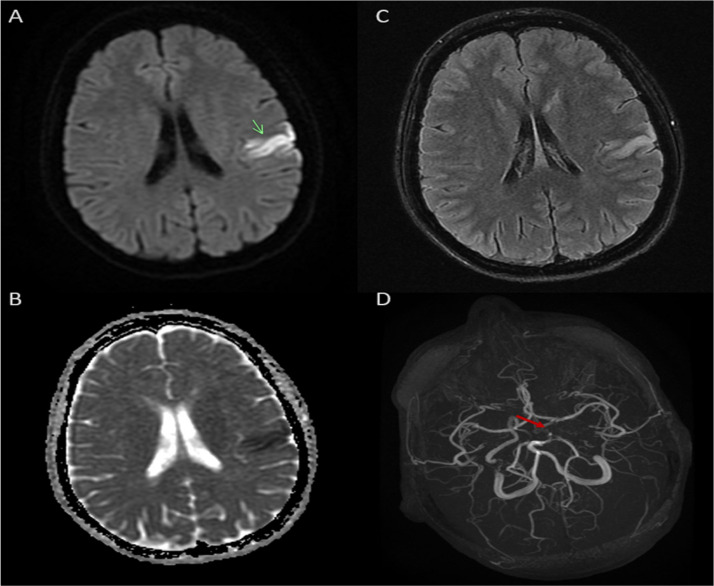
Brain MRI at presentation. (A) Axial DWI shows a focal cortical hyperintense lesion in the left fronto-parietal cortical region within the territory of a distal cortical branch of the middle cerebral artery MCA (green arrow). (B) ADC map demonstrates corresponding hypointensity, confirming true diffusion restriction. (C) Axial FLAIR sequence shows subtle matching cortical hyperintensity without mass effect or hemorrhagic transformation. (D) TOF-MRA reveals asymmetry of the anterior circulation, with reduced opacification and caliber of the left carotid/MCA inflow and irregular signal at the terminal ICA–A1/M1 junction (red arrow), suggesting hemodynamic compromise of the left ICA pathway with collateral compensation.
